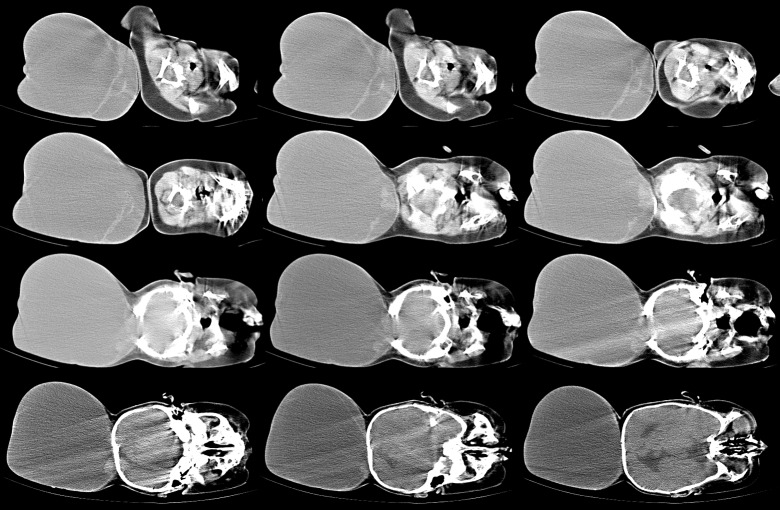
Figure 2: CT scan brain showing large encephalocele sac with protrusion of the contents.
